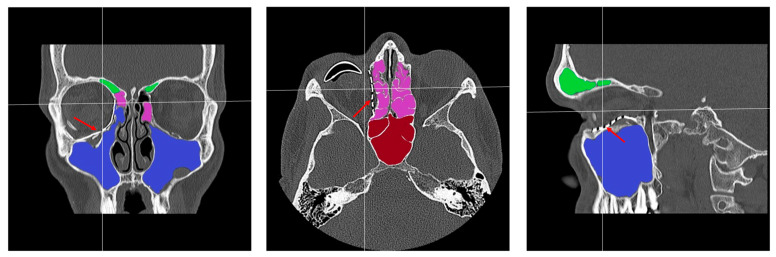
Post-operative CT (example) with colored PNS (green—frontal, violet—anterior and posterior ethmoid, magenta—sphenoid, blue—maxillary sinuses) and PSI in situ (red arrows).
